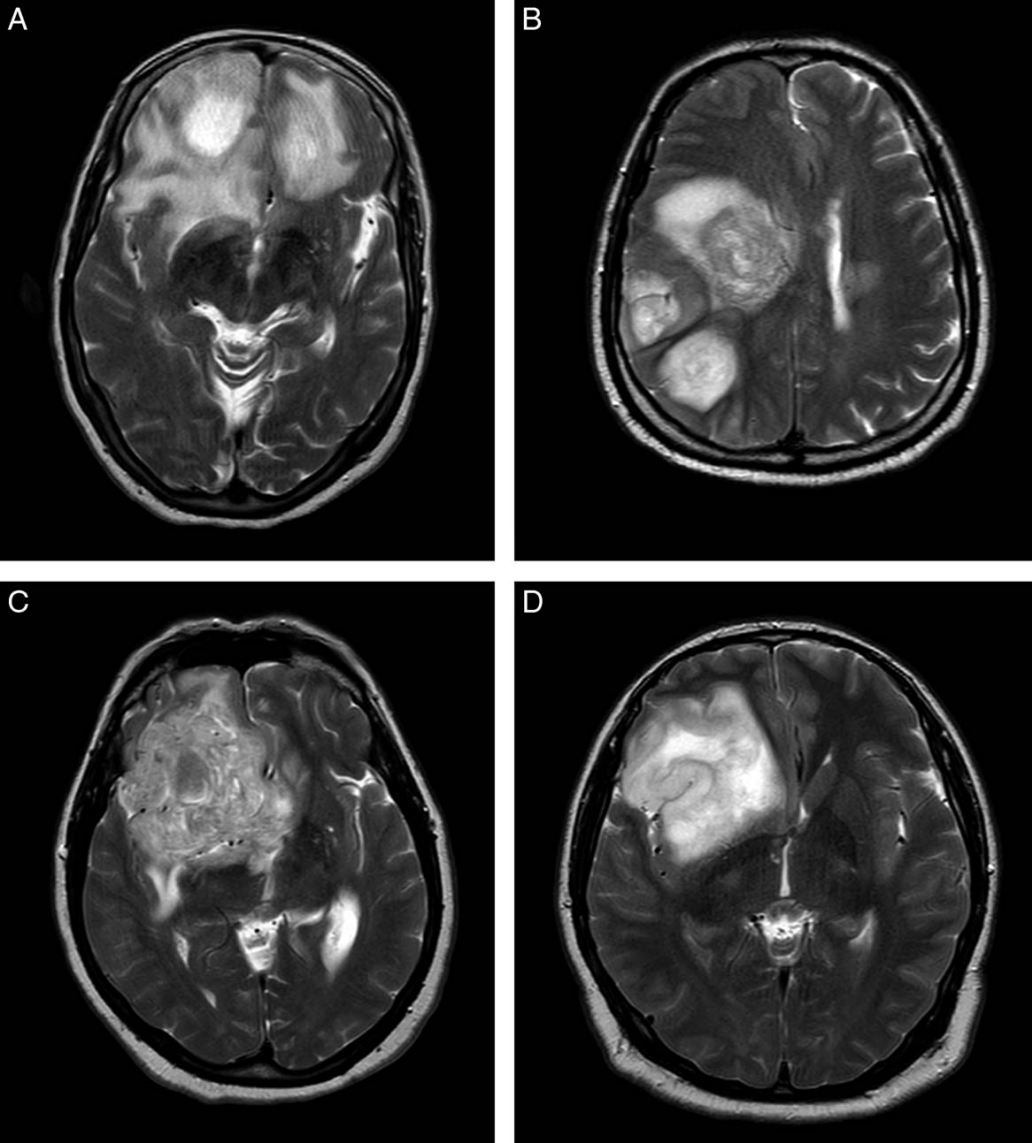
Axial T2-weighted MRI images of glioblastoma multiforme (GBM) in four patients: (A) Multi-focal GBM lesions in the right and left frontal lobes of an 81-year-old female (patient 1). (B) Multi-focal intracranial GBM lesions in a 62-year-old male (patient 2). (C) A right lateral ventricular and right frontal lobe GBM with mass effect in a 63-year-old female (patient 3). (D) A right frontotemporal GBM in a 32-year-old male (patient 4).
